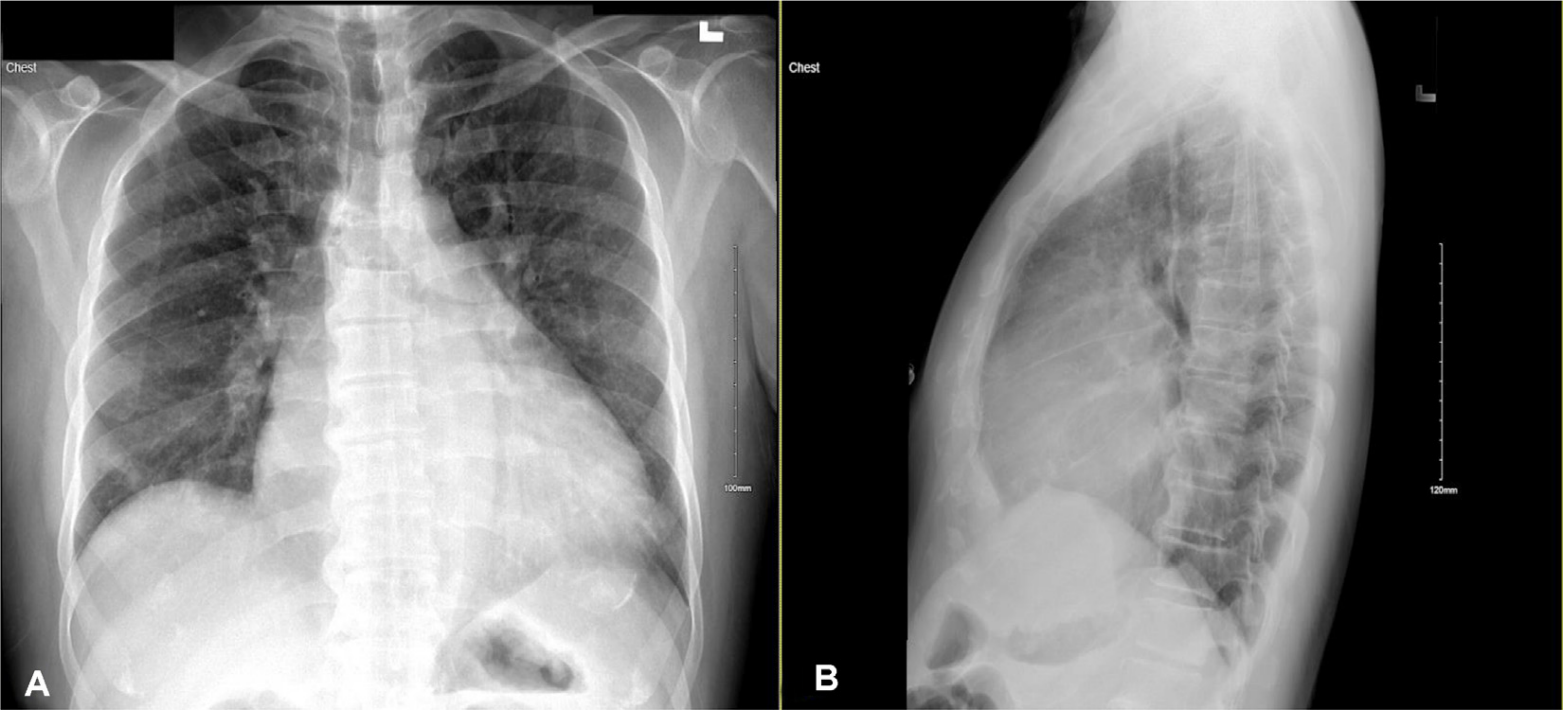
Frontal (A) and lateral (B) chest radiographs showing cardiomegaly with mild aortic uncoiling and prominent vascular markings consistent with fluid overload with mild engorgement of the margins.
